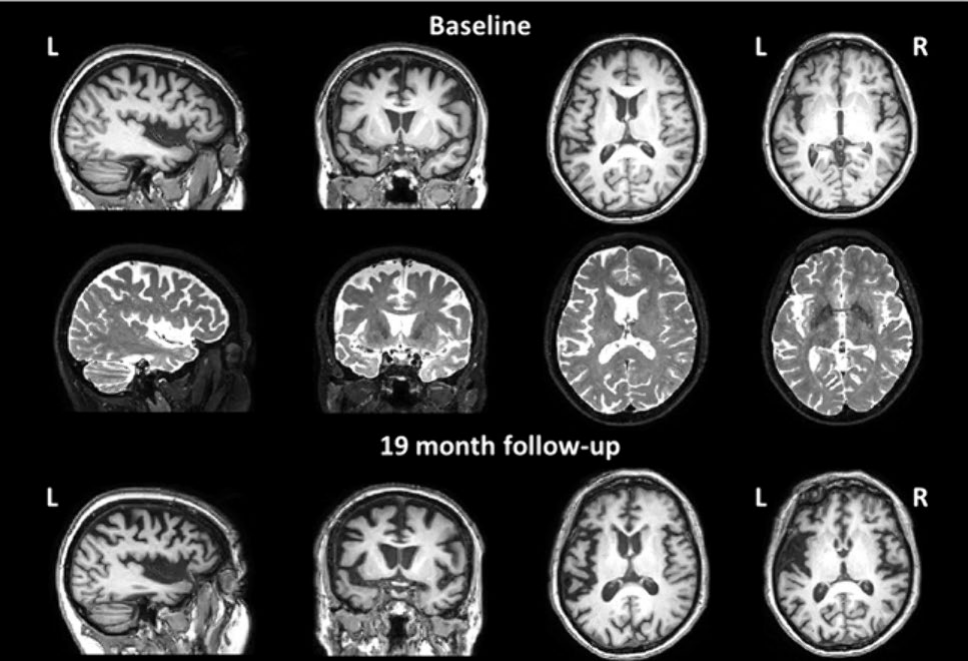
Sagittal, coronal, and axial views of the 3DT1-weighted (first row and third row) and the 3DT2-weighted (second row) image sequences of case II-3 at the first MRI scan and at 19 months of follow-up
